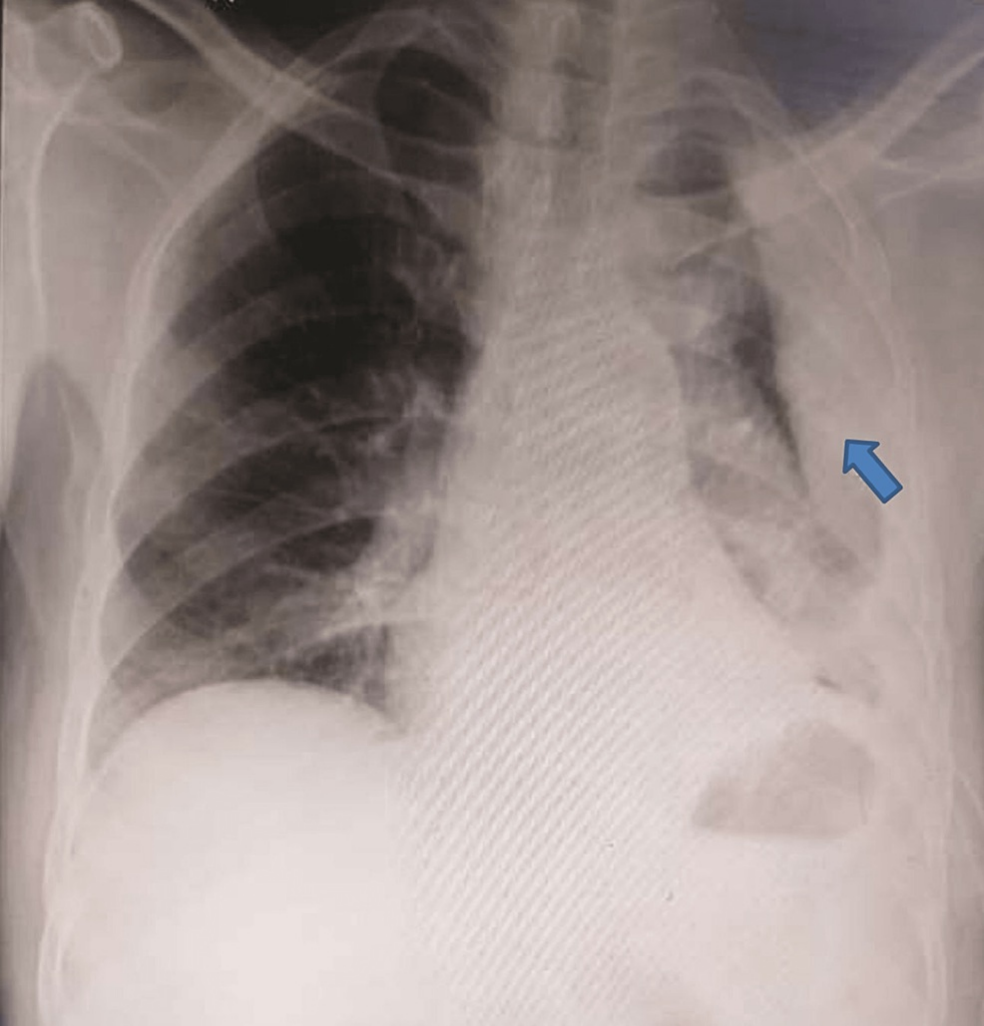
Chest X-ray of the patient. Obliteration of the left costophrenic angle with a large pleural-based dome-shaped opacity projecting into the lung was detected, with tracking along the costophrenic angle and lateral chest wall, indicating loculated pleural effusion.
